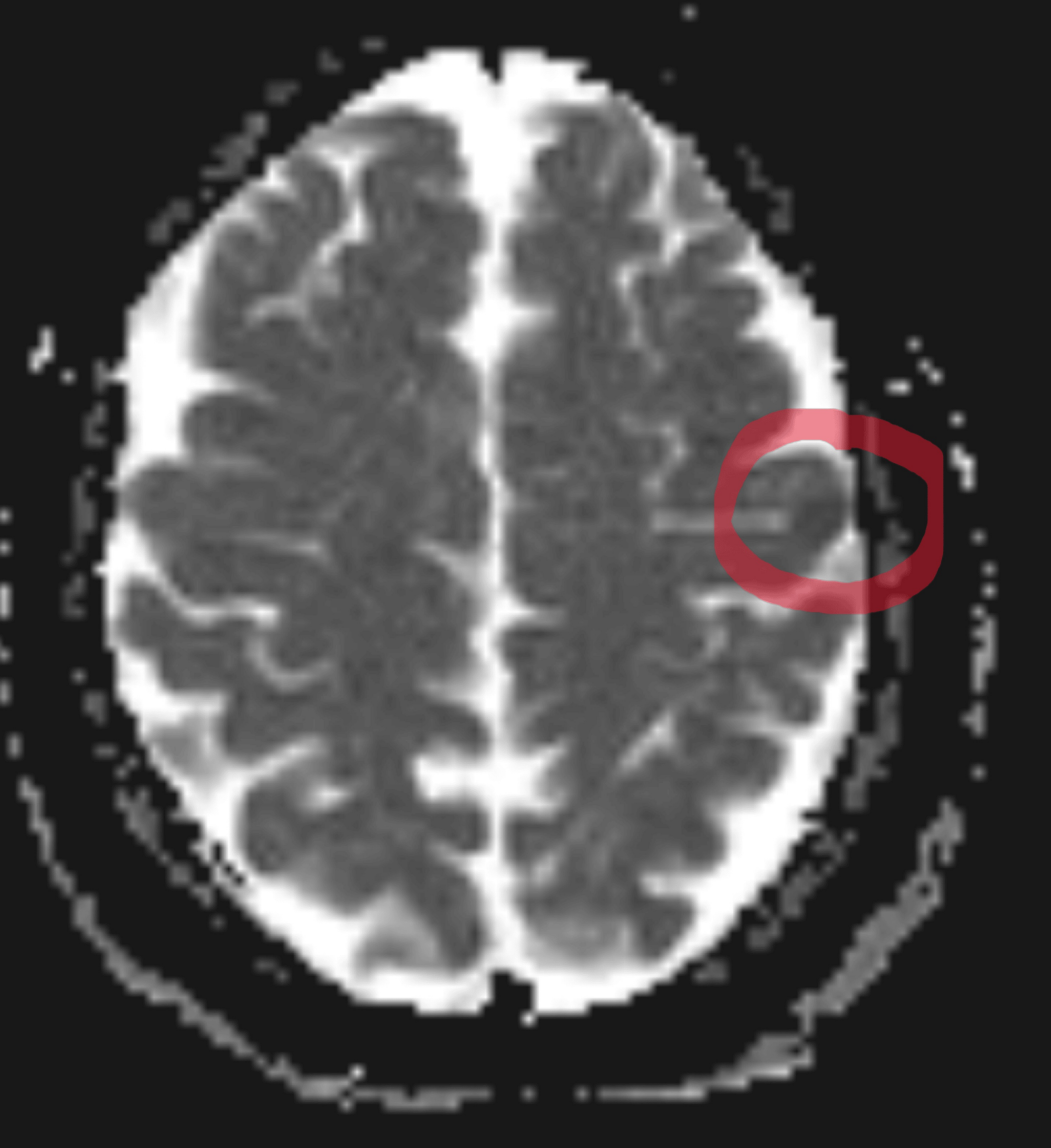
MRI ADC sequence showed an area of hypoperfusion at the same location of the DWI abnormality ADC: Apparent diffusion coefficient; DWI: diffusion-weighted imaging
